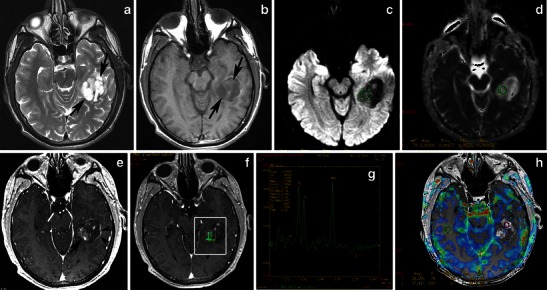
Hemispheric pilocytic astrocytomas in a 26-year-old man. a An axial T2-weighted image shows a temporally located, lobular mass that is very hyperintense (arrows) without vasogenic oedema. b On this T1-weighted image, the lesion is hypointense compared with grey matter (arrows). c The lesion exhibits low signal on diffusion-weighted imaging, with high signal intensity on an ADC map (d). e On this post-contrast, T1-weighted image, nodular (arrow) and peripheral rim enhancements (arrowheads) are visible. f, g MRS with TE 144 ms revealed an elevated choline peak without reduction of the NAA peak. h Perfusion MRI reveals low rCBV in the solid (enhanced) part of the tumour
